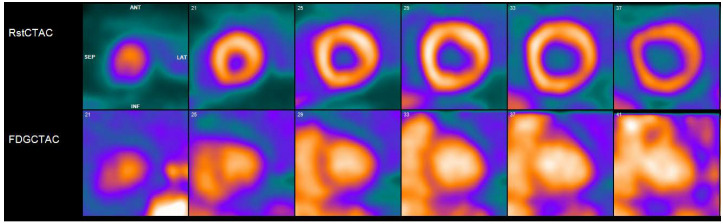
Cardiac positron emission tomography/computed tomography (PET/CT) with ^13^N-ammonia (top row) and ^18^F-flurodeoxyglycose (FDG) (bottom row) demonstrates normal resting perfusion and adequate myocardial suppression with no evidence of FDG uptake to suggest active inflammatory state.
